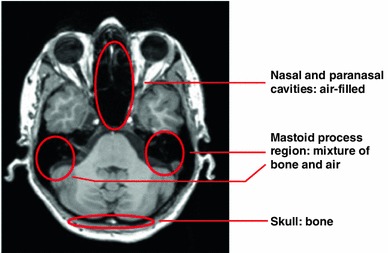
The T1-weighted MR image slice shows an air-filled nasal cavity, the mastoid process as a mixture of lamellar bone and small cavities, and the skull. The *dark areas* representing bone and air appear in the same intensity range
